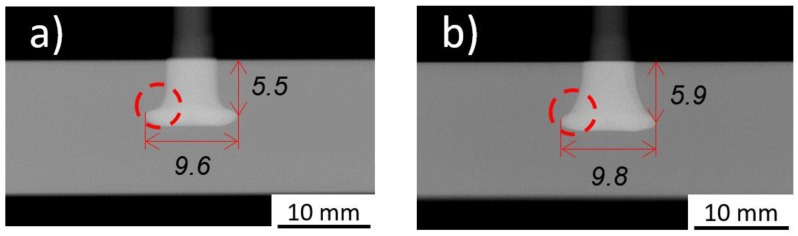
X-ray tomography of: (a), Condition 14 (RS = 20,000 rpm; FT = 1.6 s; FoT = 2 s; FF = 3000 N; FoF = 3900 N); and (b), Condition 36 (RS = 19,000 rpm; FT = 1.8 s; FoT = 1.5 s; FF = 2500 N; FoF = 5700 N).
